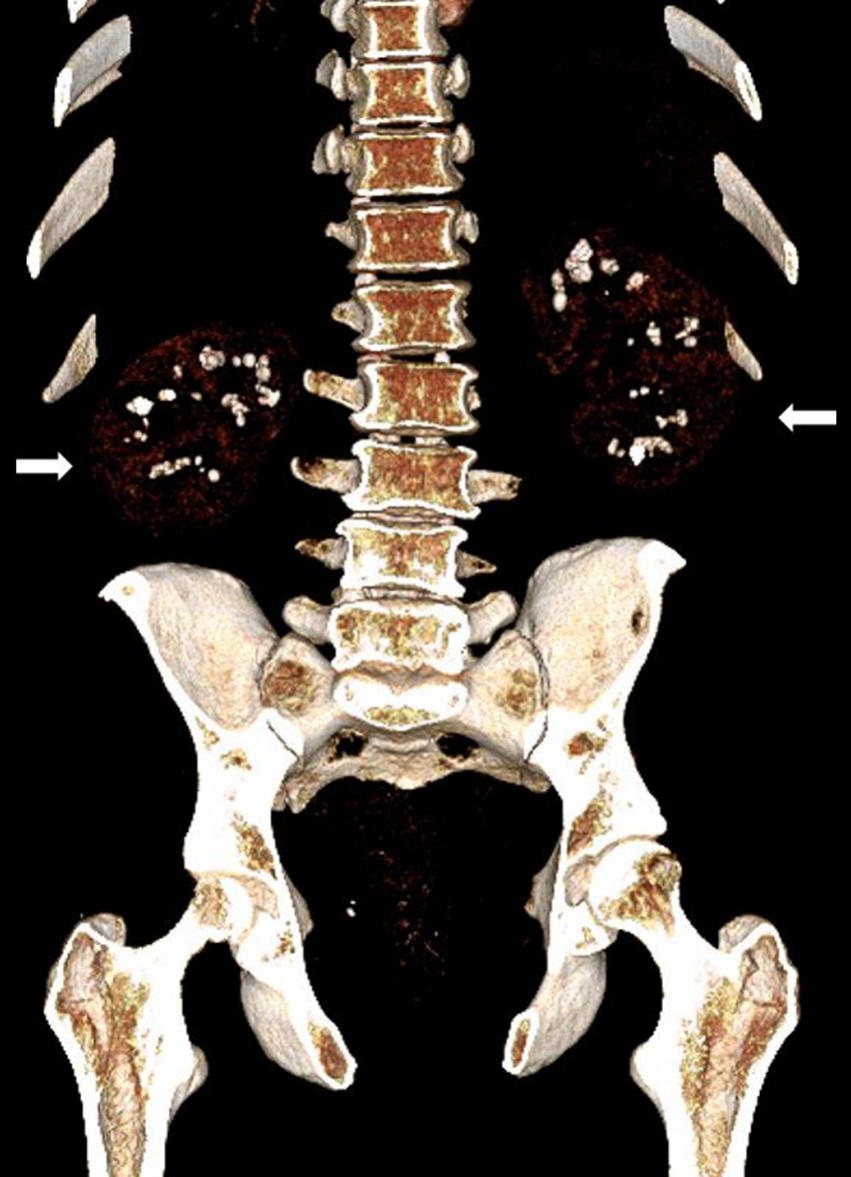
Three‐dimensional reconstruction of the CT scan demonstrating bilateral medullary nephrocalcinosis.
